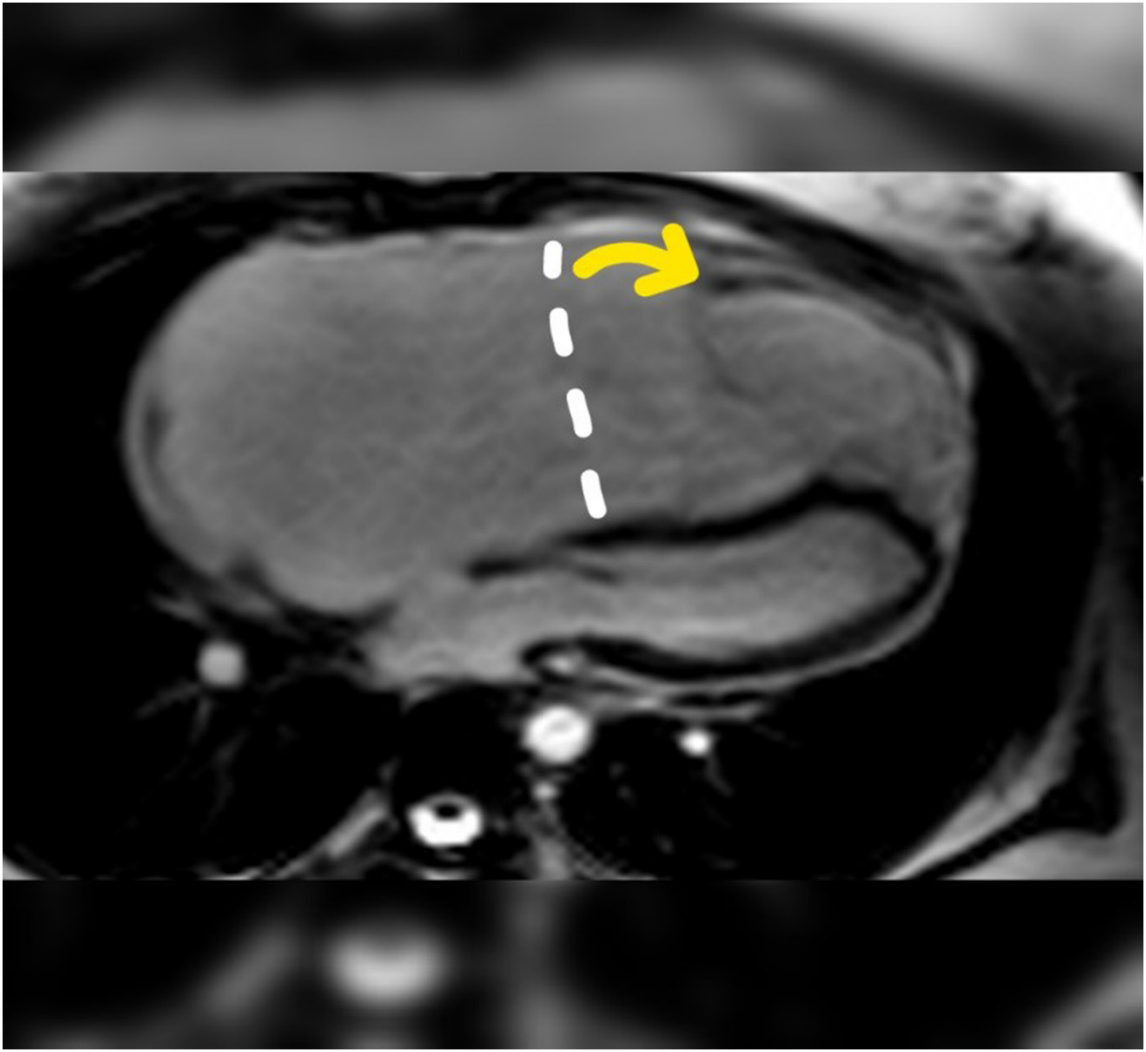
Apical displacement of tricuspid anterior leaflet (5.3 cm, yellow arrow) relative to tricuspid annulus in cardiac magnetic resonance imaging.
